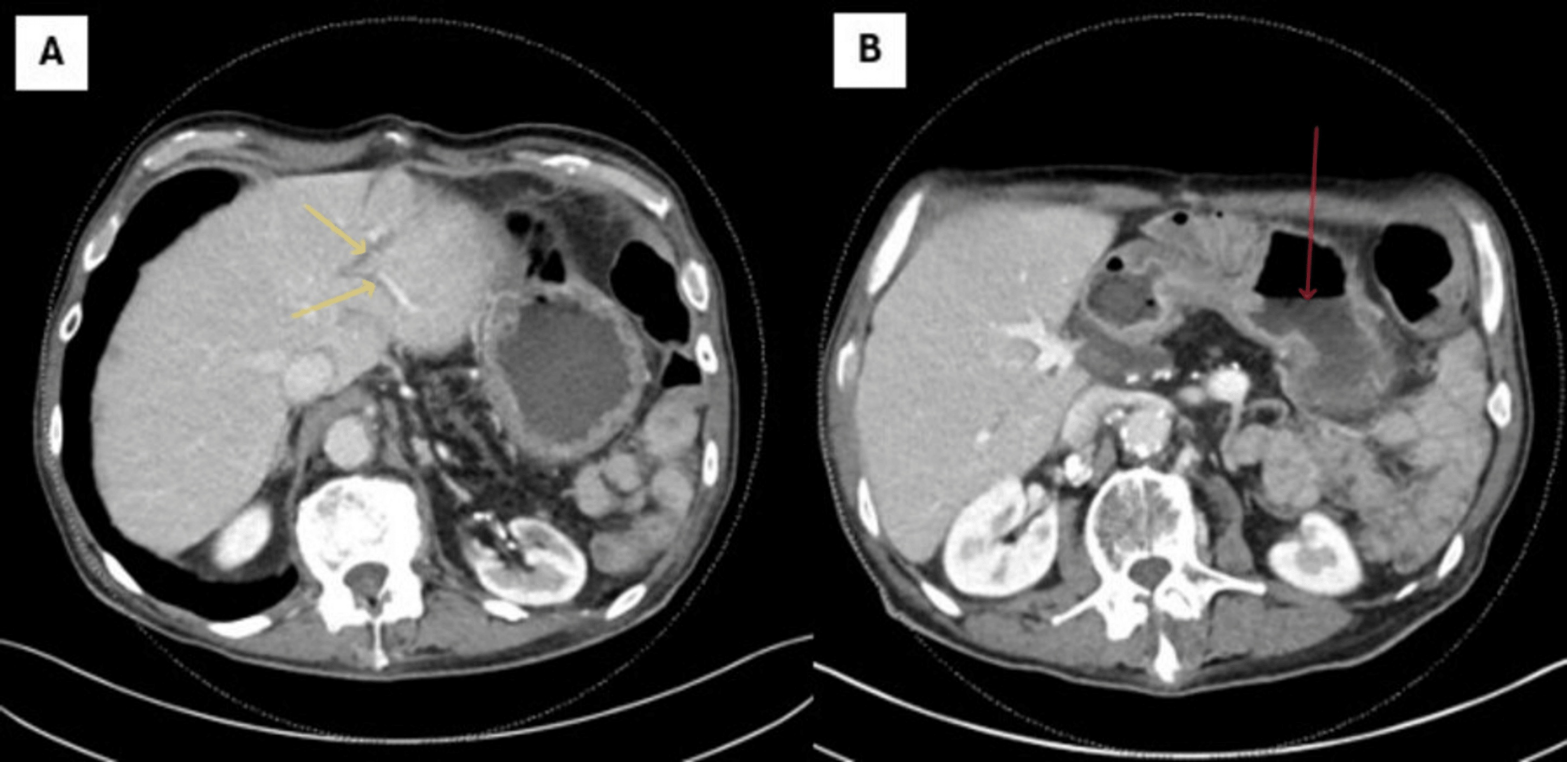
A) New mildly dilated intrahepatic bile ducts on axial CT of the abdomen. B) Retained gastric content and air fluid level Yellow arrow: dilated intrahepatic ducts; Red arrow: retained gastric contents
